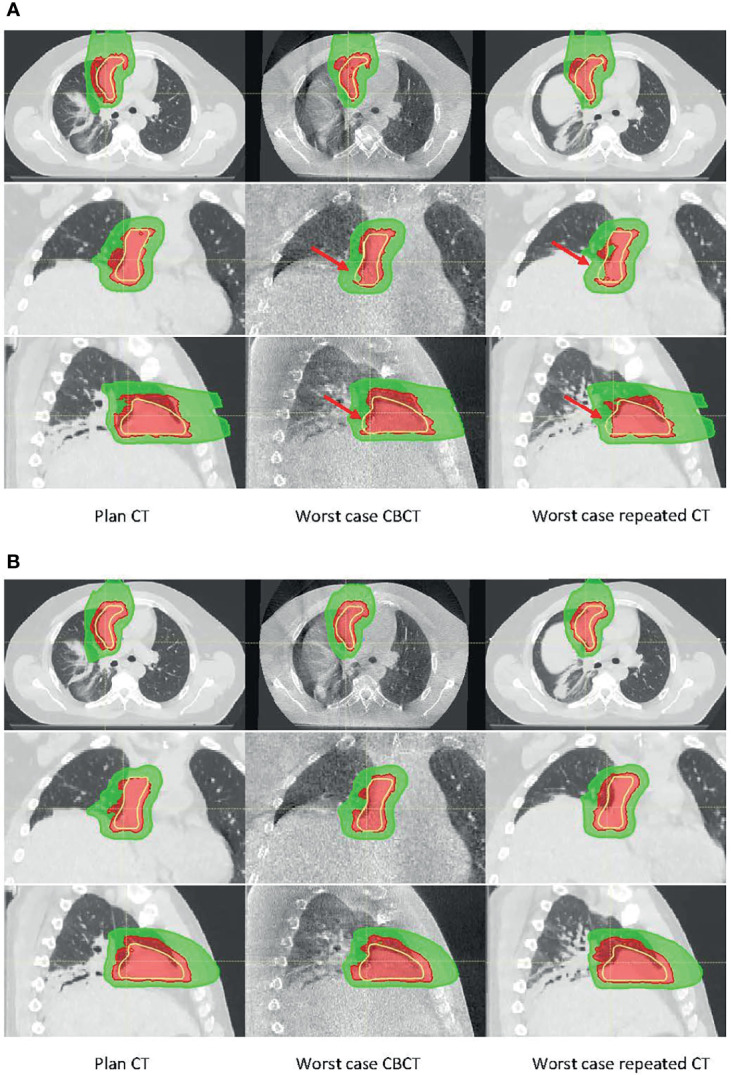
Dose distribution calculated on planning CT (left), worst-case cone-beam CT (CBCT) (middle), and worst-case repeated CT (right) for (A) the single breath-hold BH CT plan and (B) multiple BH CT plan. There were ~8-mm superior–inferior (SI) BH uncertainties between the planning CT and the worst-case CBCT or CT. Red arrows in panel A point to the area where target coverage was reduced.
